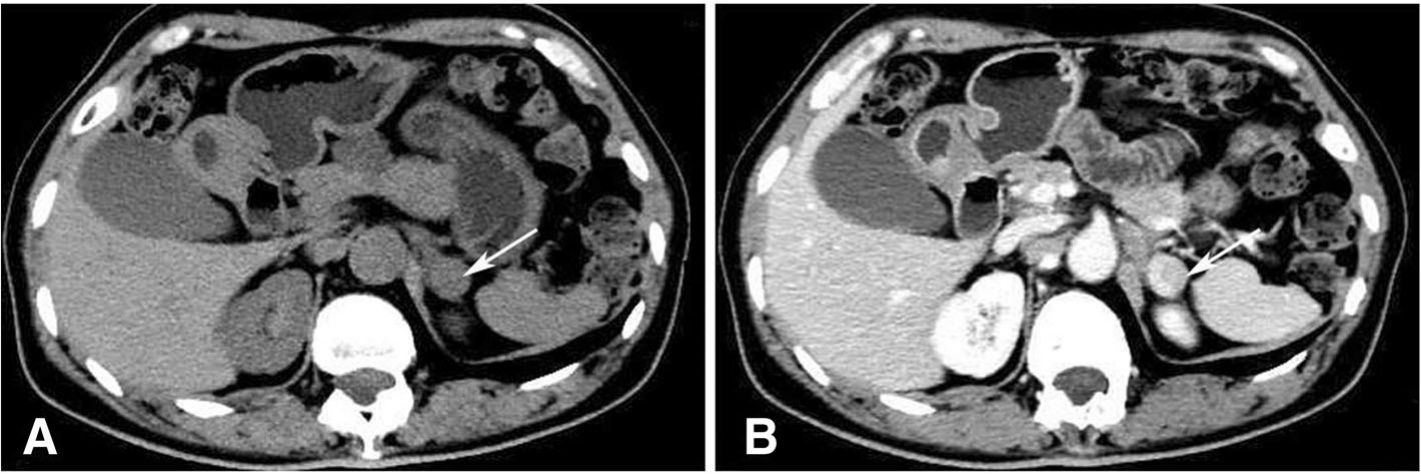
Adrenal CT (a) and enhanced CT (b) identified a left adrenal mass, 1.5 cm in diameter (Arrow). CT = computed tomography
